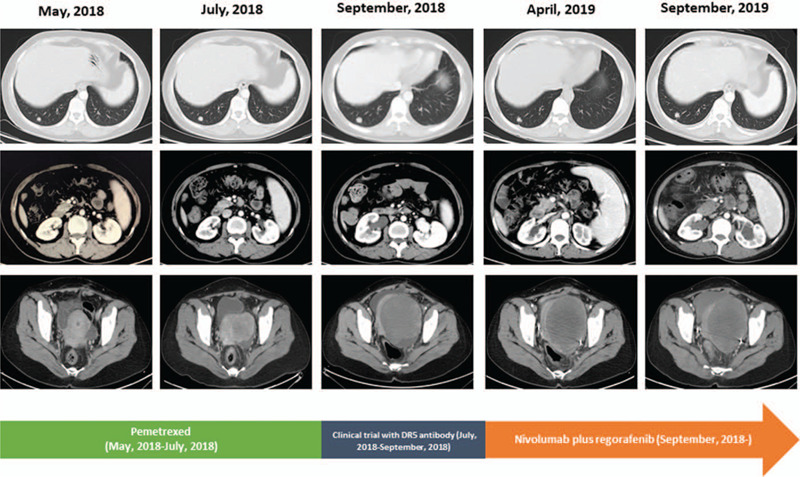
computed tomography images showing the changes of masses in lung, abdomen, and pelvis during treatment course.
